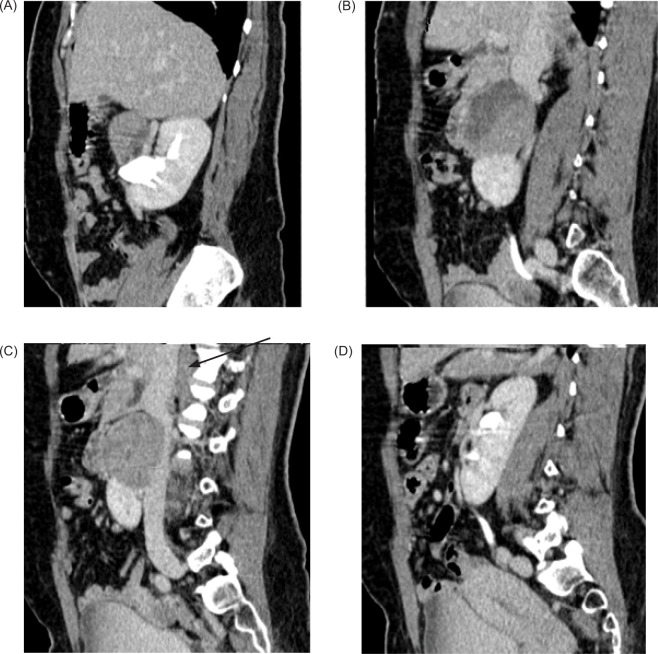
(A–D) Contrast enhanced CT sagittal images—Mass arising from the isthmus, posteriorly compressing the IVC with obliteration of intervening fat planes (arrow).
